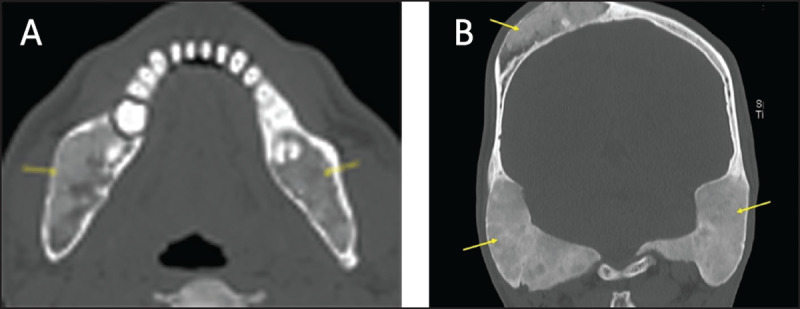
(A, B) Polyostotic fibrous dysplasia. Axial (a) and coronal (b) CT showing fibrous dysplasia lesions with typical ground glass appearance in the mandibles and skull bones (arrows).
